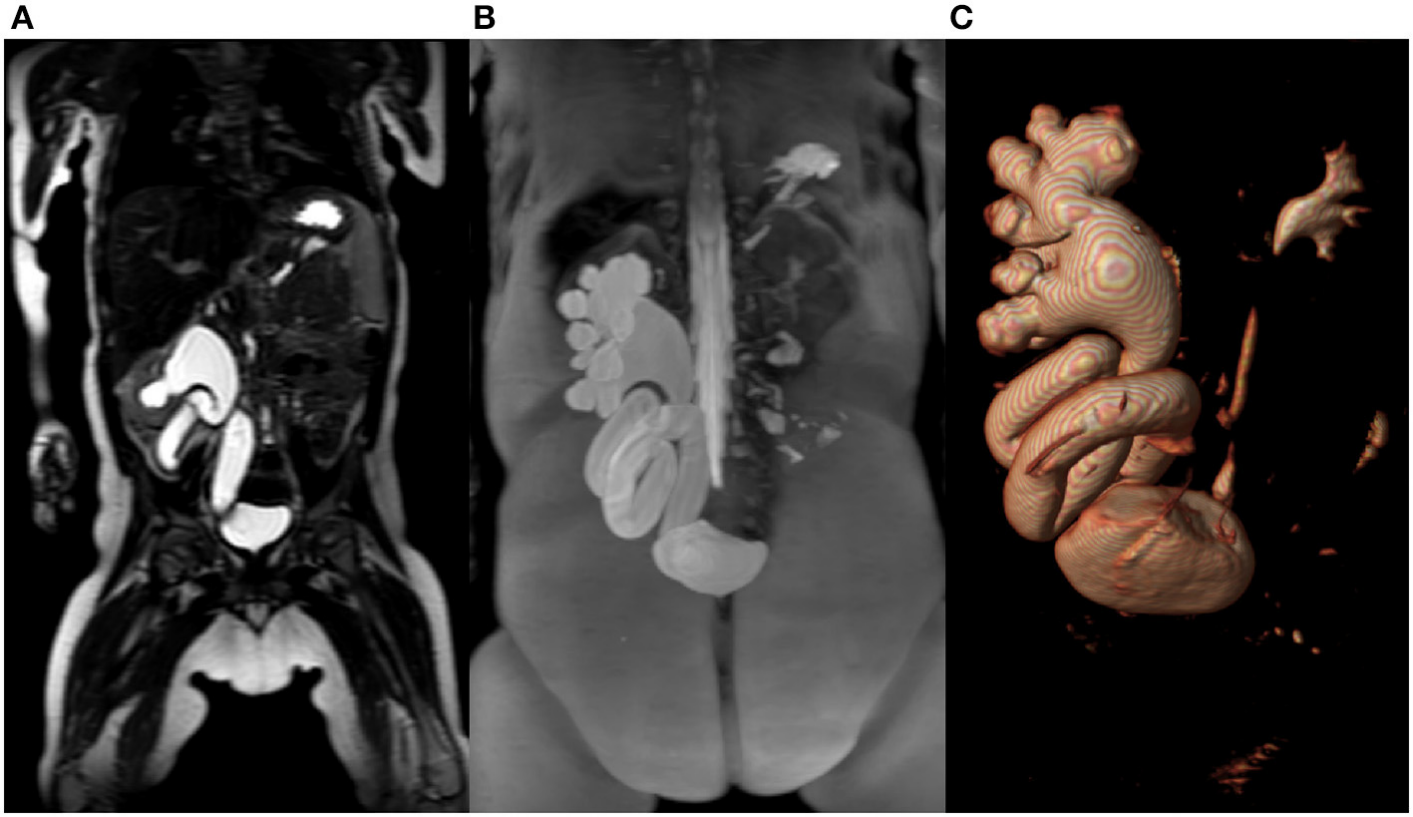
(A) T2-weighted- image in coronal plane of right kidney with megaureter. (B) Maximum intensity projection (MIP) reconstruction image of the right kidney collecting system with megaureter. (C) The same patient with reconstruction in 3D-volume rendering technique.
